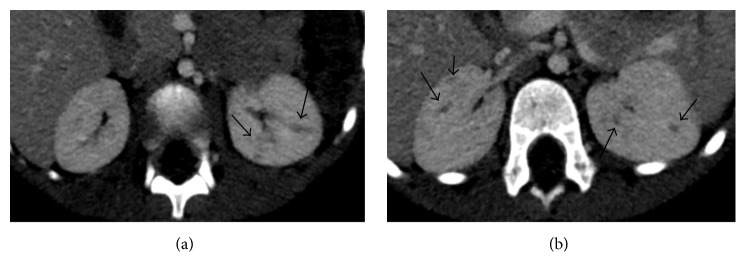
Two axial contrast-enhanced CT images through the level of the kidneys demonstrate small bilateral hypoattenuating renal lesions.
